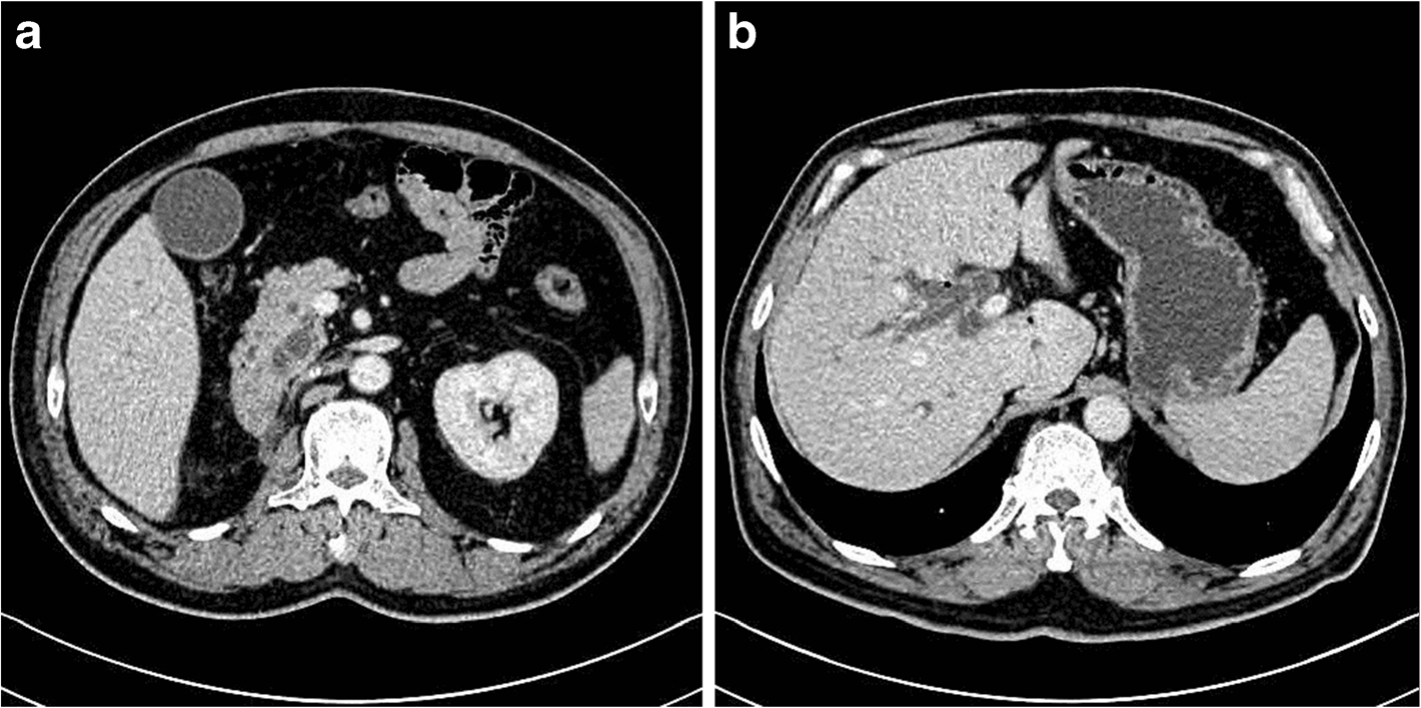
Computed tomography (CT) scan results showing (a) obstruction of the lower biliary tract and right kidney deficiency and (b) the intrahepatic bile duct dilatation.
